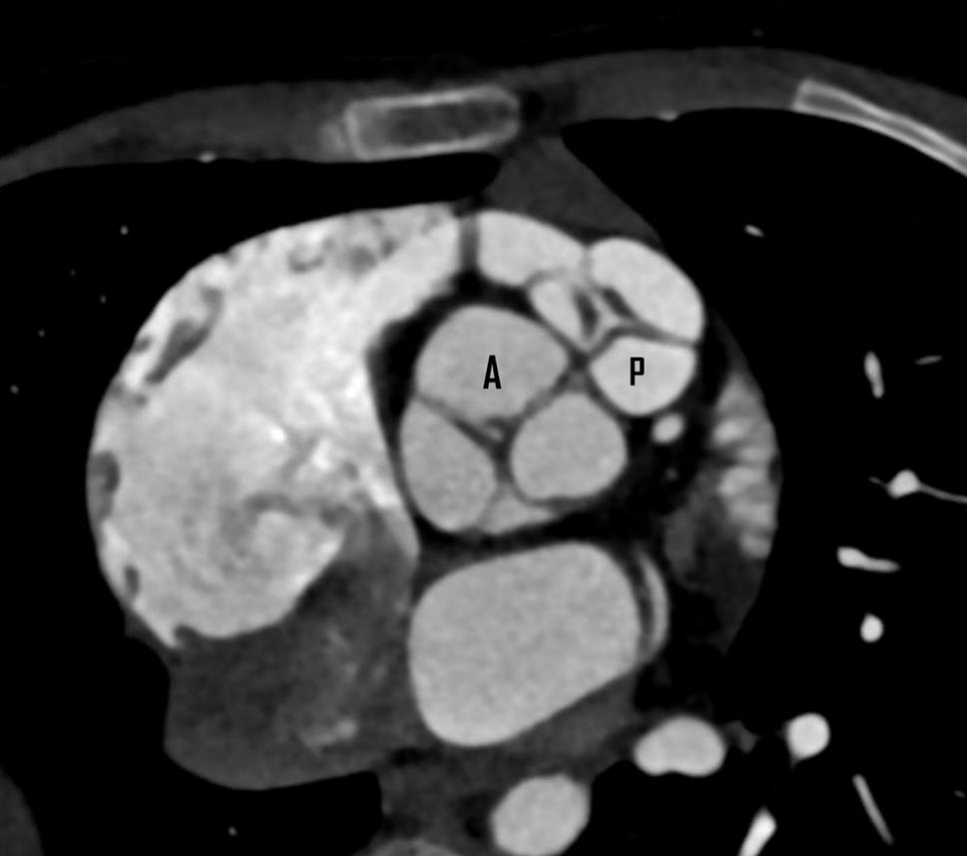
Cardiac CT double oblique image showing thickened aortic valve (A) and pulmonary valve (P) leaflets
